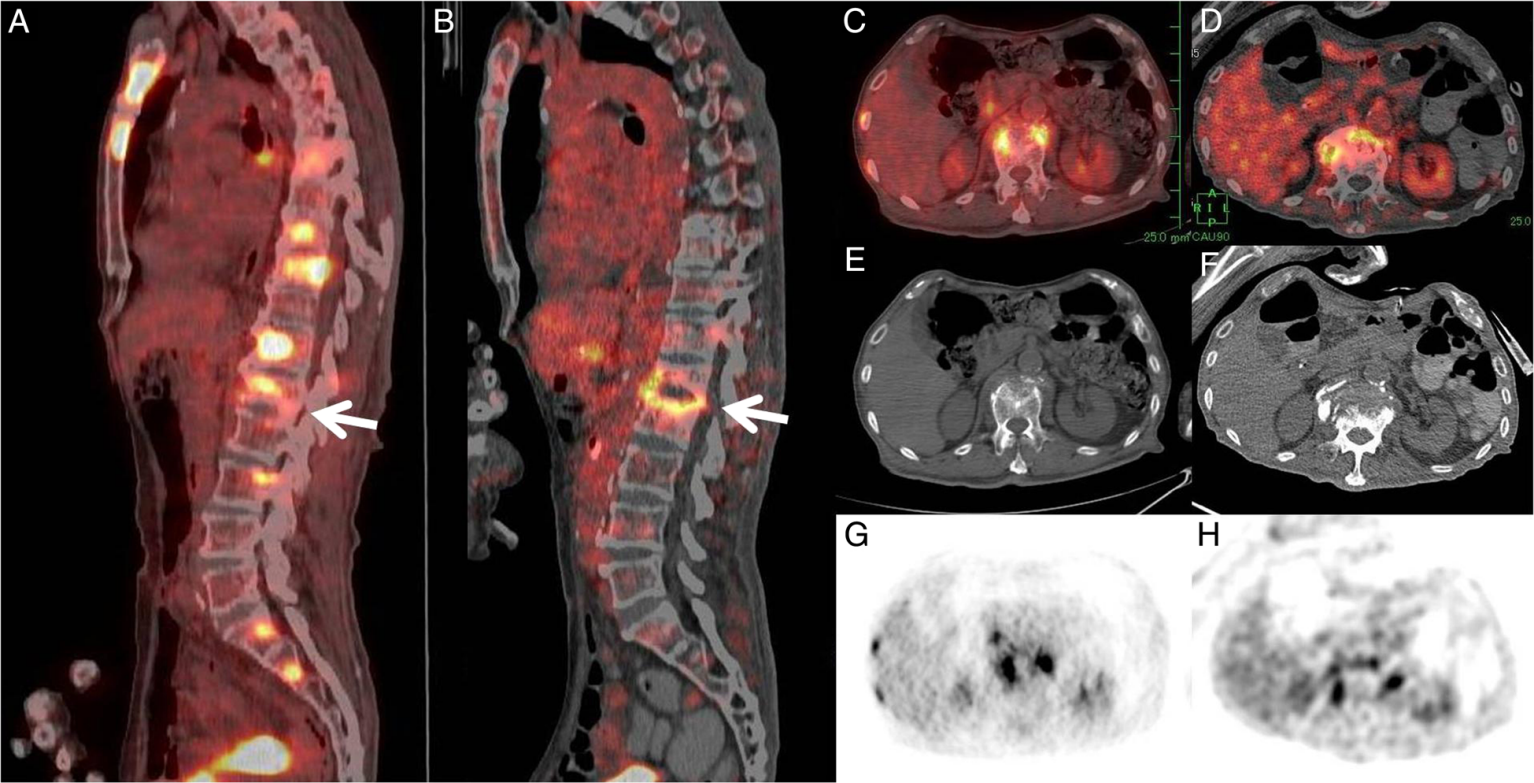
Multifocal bone and lymph node tuberculosis with concomitant psoas abscess demonstrated on positron emission tomography-computed tomography (PET/CT). The sagittal (A, B) and transverse (C, D) PET/CT fusion images, theaxial unenhanced CT images (E, F) and the FDG-PET images (G, H) showed persistent FDG uptake corresponding to the L1 vertebral body destruction (arrow) and abscesses in the bilateral psoas major muscles before (A, C, E, G) and after psoas abscess drainage and 9 months of antituberculous medications (B, D, F, H).
